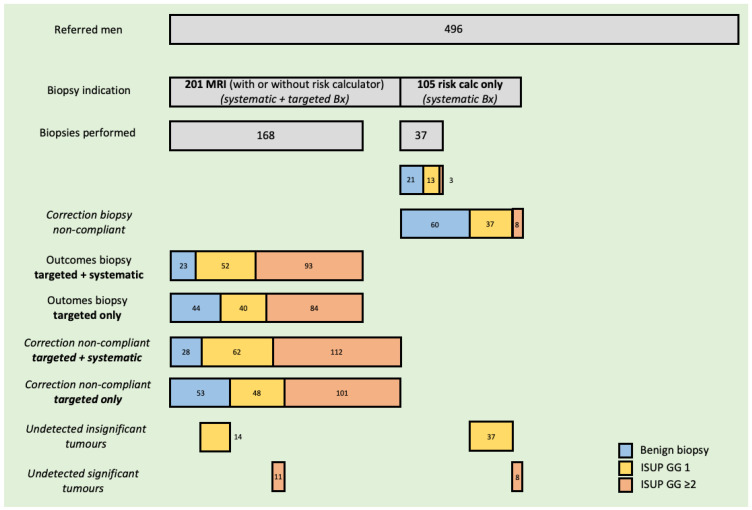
Extrapolated to 100% biopsy indication compliance, 120 (8 + 112) significant and 99 (37 + 62) insignificant tumors would have been detected. Omission of systematic biopsies in cases with normal MRI (PIRADS 1–2) but abnormal risk-calculator (≥20%) would miss 7% (8/120) of significant and 37% (37/99) of insignificant tumors. Targeted biopsy only and omission of systematic biopsies in cases with abnormal MRI (PIRADS 3-4-5) would miss 9% (11/120) of significant and 14% (14/99) of insignificant tumors.
